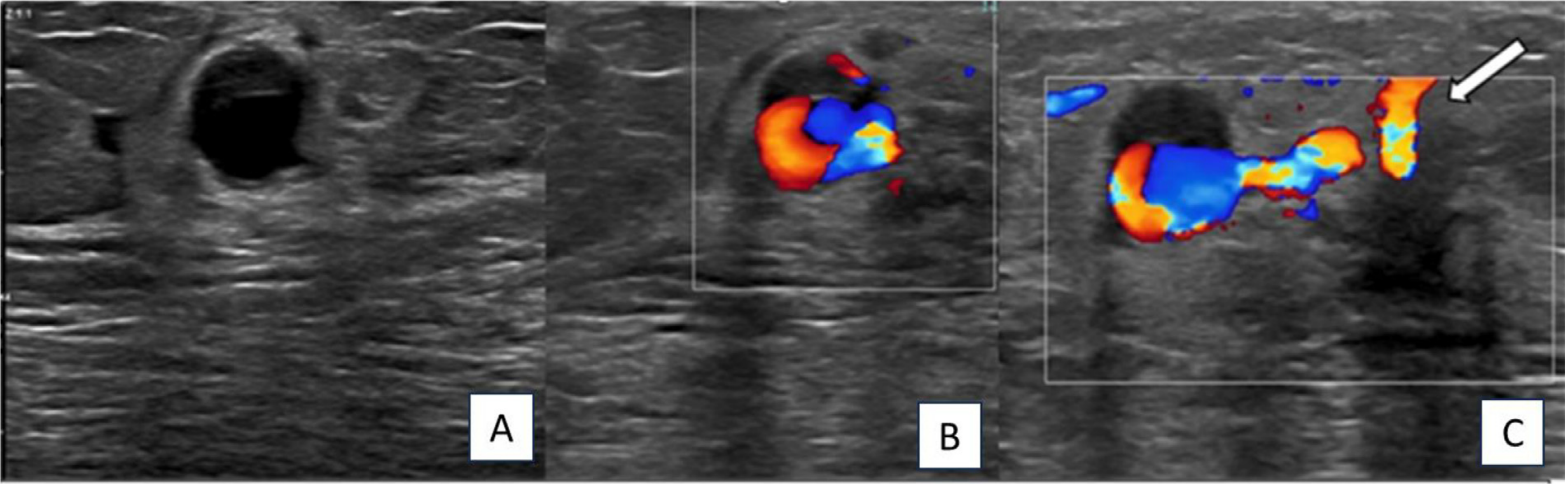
Axila greyscale ultrasound examination shows rounded anechoic mass at the site of prior biopsy area (image A), medial to the piopsy proven malignant mass. Color Doppler shows blood flow inside the mass with characteristic “yin-yan” sign of pseudo aneurysm (image B). Note associated adjacent artery (white arrow in image C).
